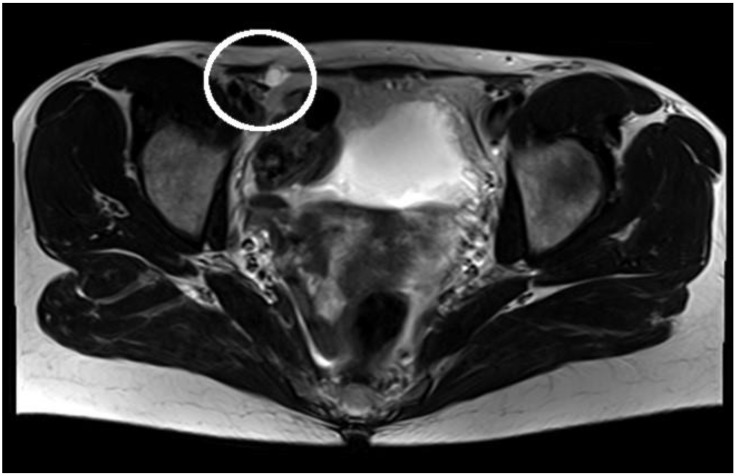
Axial T2-weighted MRI: cystic structure localized in the right inguinal canal, with no communication to the peritoneal cavity.
